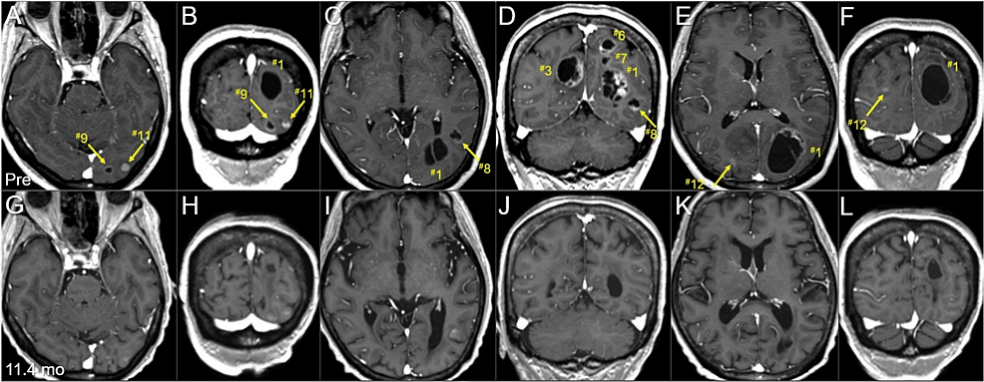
Magnetic resonance images at the diagnosis and last examination, focusing on the four non-boost lesions. The images show CE T1-WIs (A-L); axial images (A, C, E, G, I, K); coronal images (B, D, F, H, J, L); three days before the initiation of WBRT (pre) (A-F); and 11.4 months after the WBRT initiation (G-L). (A-L) These images are shown at the same magnification and coordinates under co-registration and fusions based on the pre-irradiation images. (A-F) Eight lesions are observed, most of them cystic, with some solid components (^#^1,3,6). Two small lesions (^#^11,12) are mainly solid. (G-L) At 11.4 months, all the four non-boost lesions (^#^8,9,11,12) had regressed or almost disappeared. CE: contrast-enhanced; WIs: weighted images; WBRT: whole-brain radiotherapy
